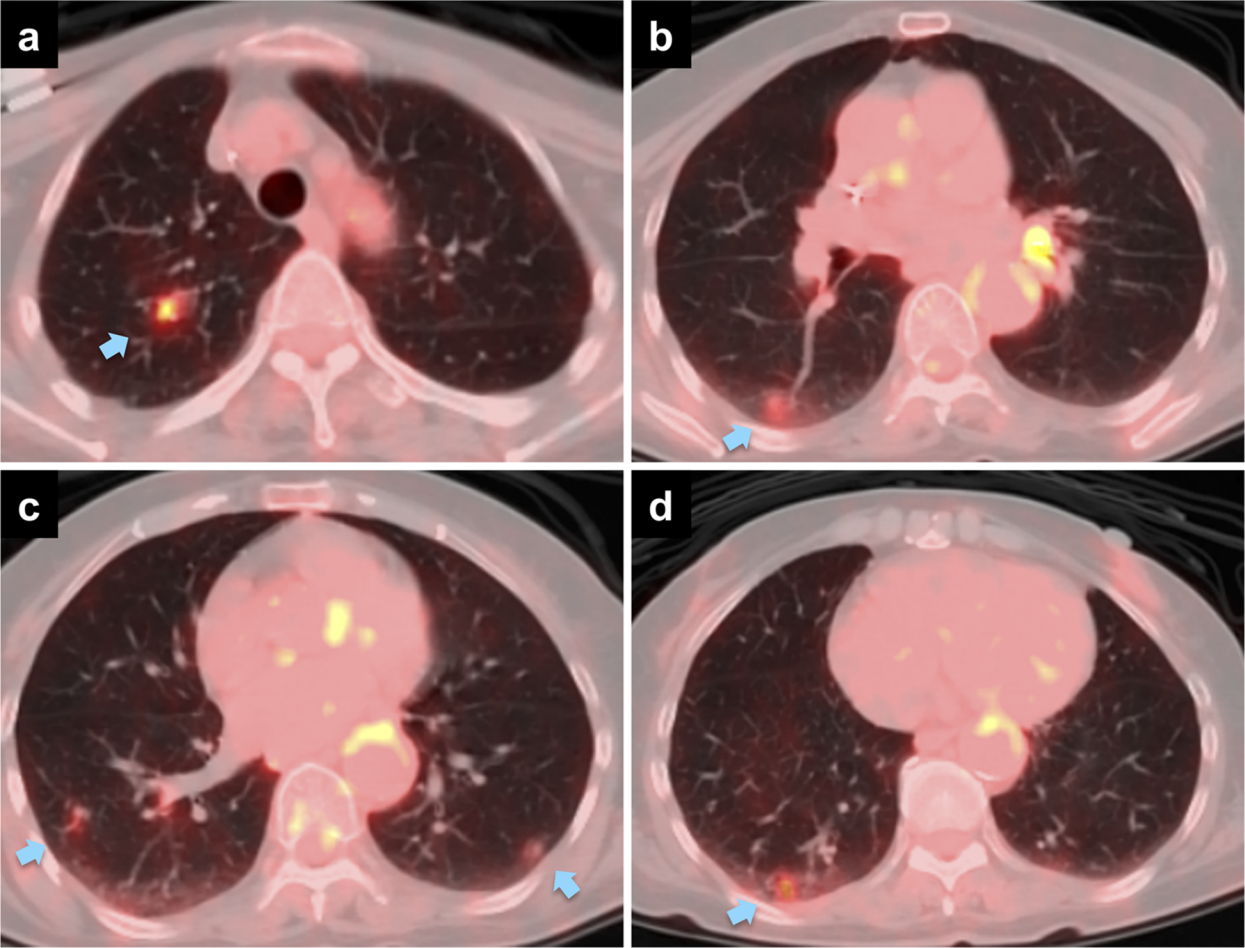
Positron emission tomography integrated with computed tomography scans. ^18^F‐fluorodeoxyglucose accumulated at the (a) right S^1^ nodule, (b) right S^6^ nodule, (c) bilateral S^9^ nodules, and (d) right S^10^ nodule (arrows).
